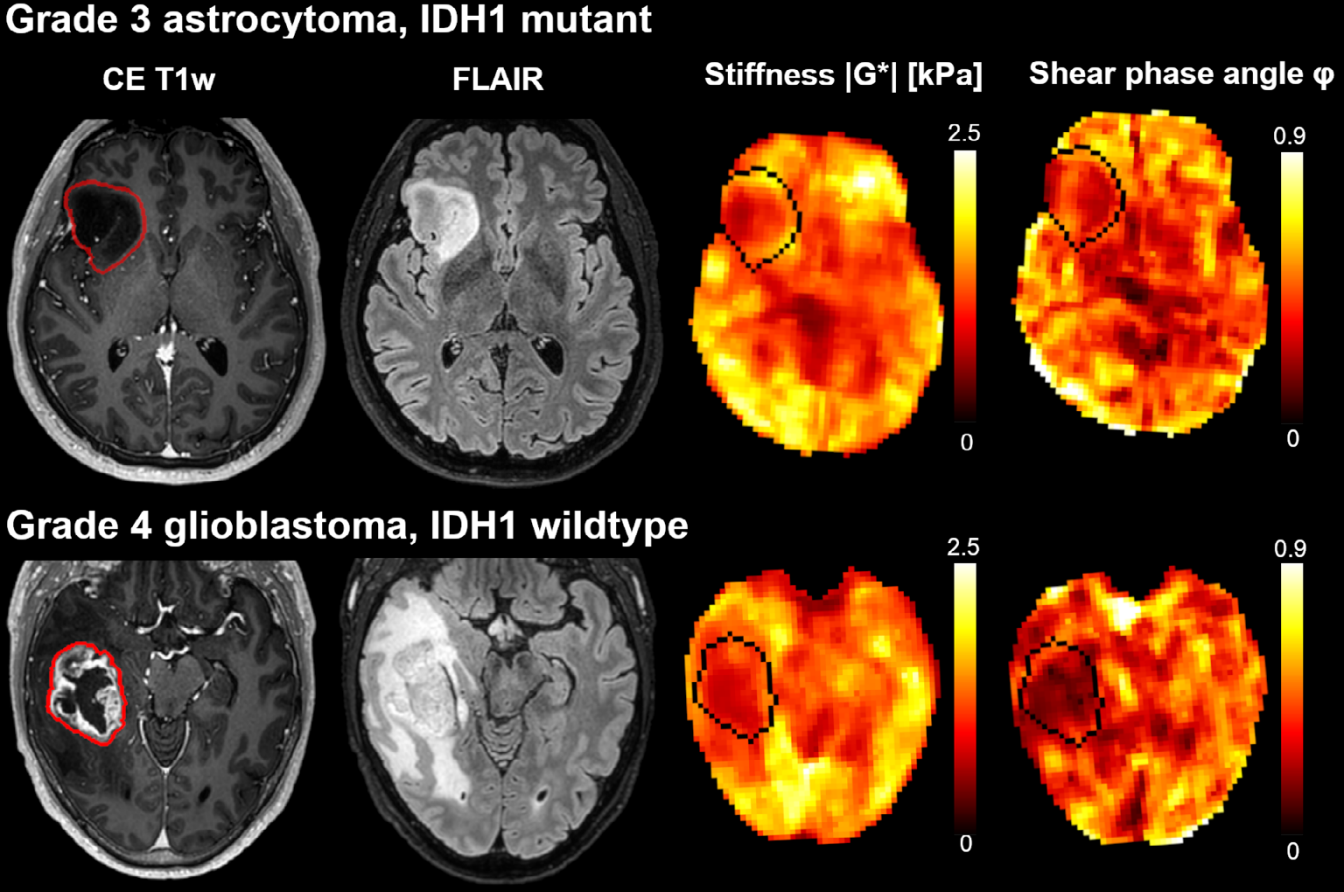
Stiffness heterogeneity of gliomas. Contrast‐enhanced T1‐weighted images, FLAIR images, |G*| (stiffness), and φ maps (phase angle, related to viscosity) for two patients with gliomas. The images in the upper row are derived from a 40‐year‐old man with an IDH1‐mutated grade 3 astrocytoma, and the images in the lower row are derived from a 55‐year‐old man with an IDH1‐wild‐type grade 4 glioblastoma.
